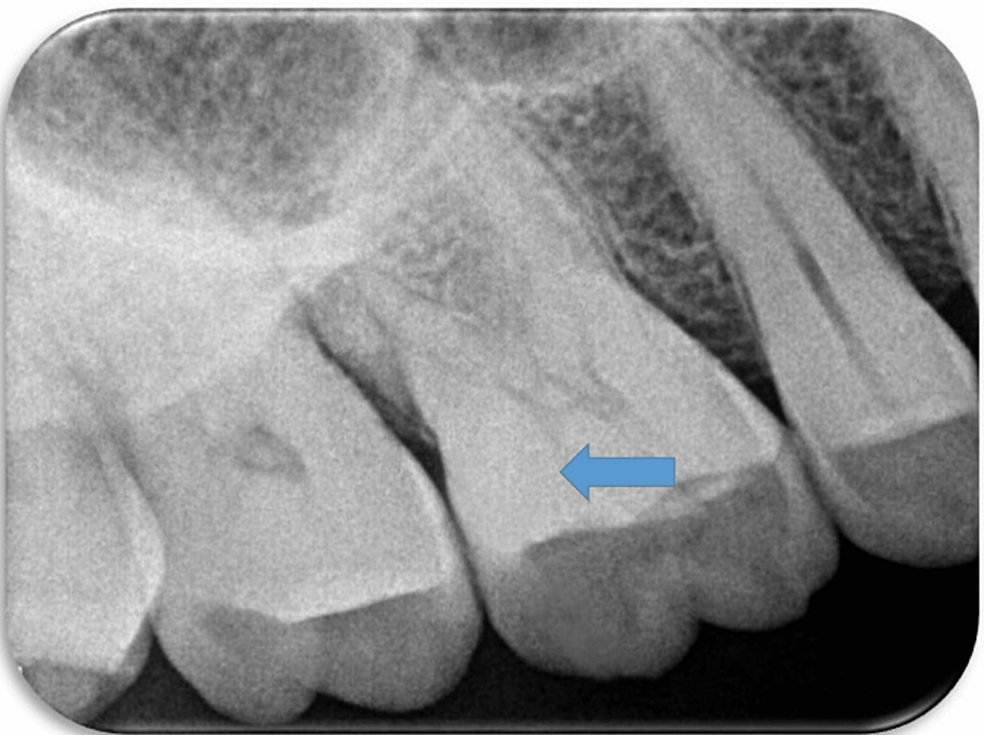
Postoperative radiographic image after onlay cementation
